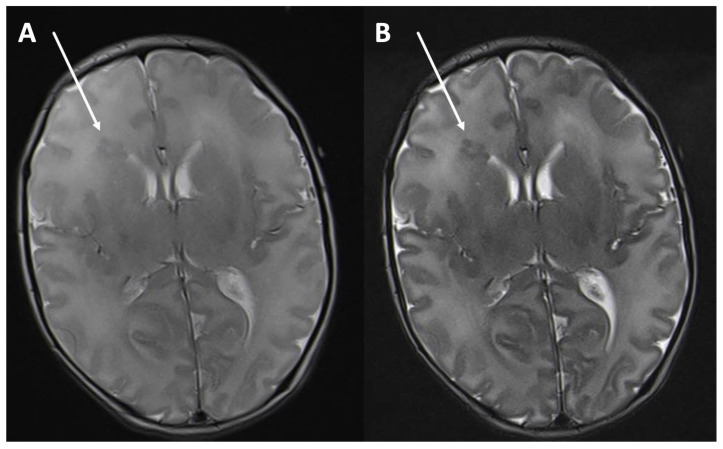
T2-weighted image in an axial orientation of a two-week-old male with seizures. The periventricular right frontal heterotopia (arrow) can be seen more clearly with a longer echo time of 203 ms, TR 5500 (B) than with a medium echo time of 113 ms, TR 5500 (A).
